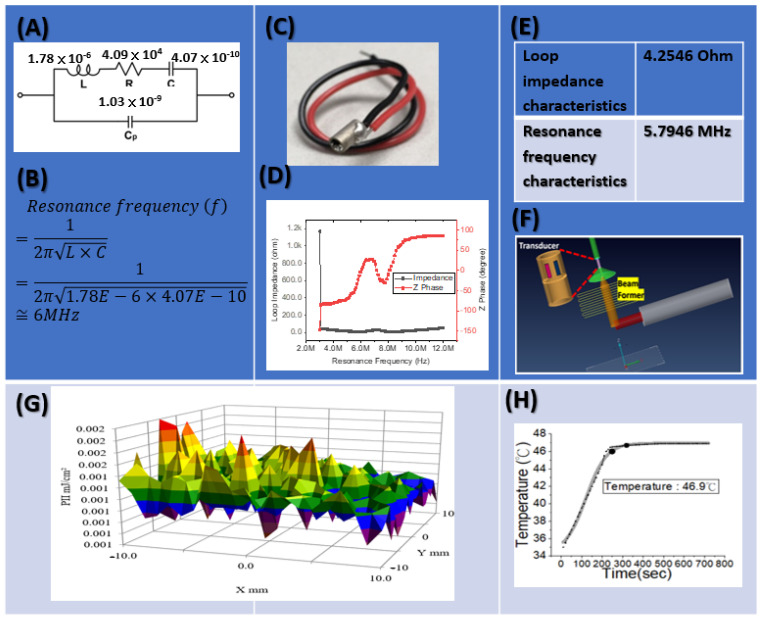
Single-channel cylindrical piezoelectric ultrasonic element (Channel A). (A) Measure resonance frequency model, The Butterworth-Van Dyke (BVD) equivalent circuit extracted data of the actual elements and calculated the equivalent resistor, inductor, and capacitor (RLC) elements. The advantage of the BVD equivalent circuit is that the circuit can be analyzed quickly. (B) Parameters, Cp: capacitance between the two electrode plates of the piezoelectric element, R: impedance is equal to R at resonance, C: capacitance is equal to C at resonance, L: inductance is equal to L at resonance. (C) Cylindrical piezoelectric ultrasonic element, (D) Loop impedance of Channel A, (E) Resonance condition, (F) Beam former scanning, (G) Energy profile, (H) Temperature observed.
